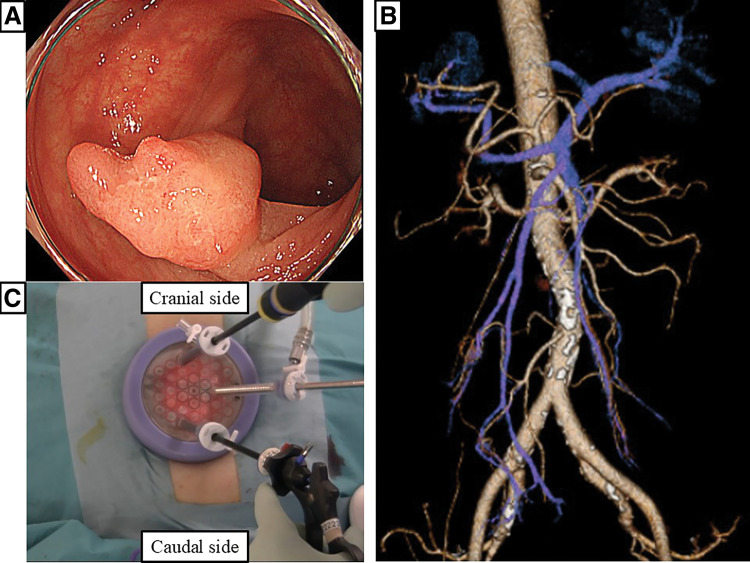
(A) Colonoscopic image: A superficial neoplastic lesion in the sigmoid colon. (B) Computed tomography angiography demonstrating complete transposition of the abdominal viscera. (C) Three 5 mm trocars inserted into the multiport access device through a 3.5 cm umbilical incision.
